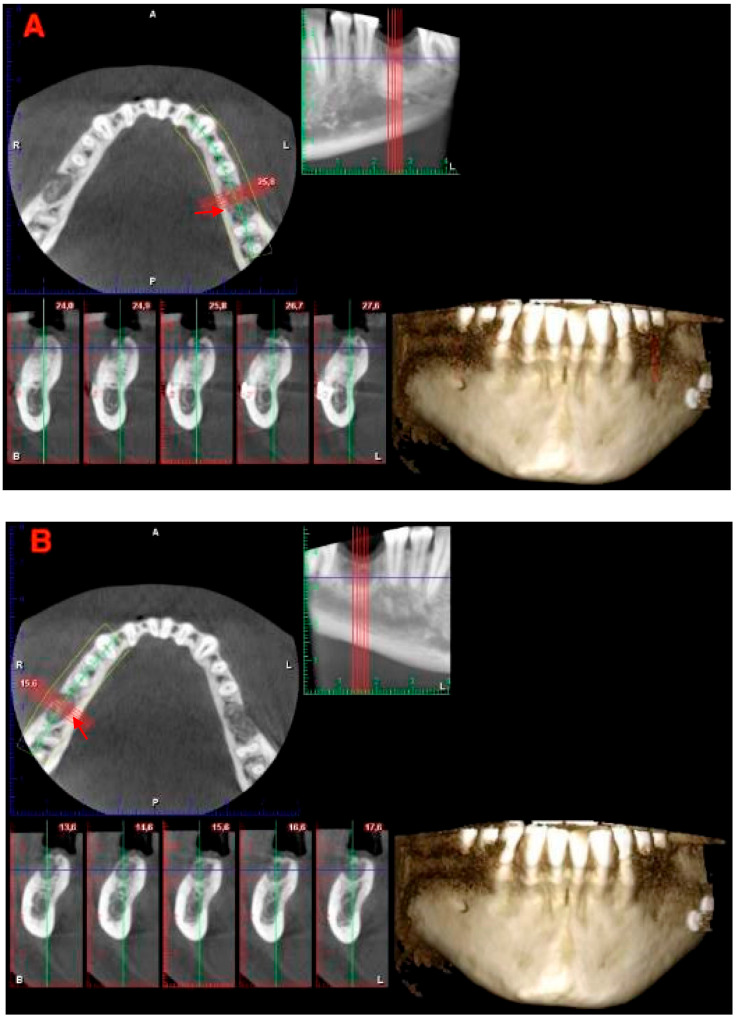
(A) CBCT at 6 months, 3.6. (B) CBCT at 6 months, 4.6. A good profile of the alveolar ridges seems to be maintained. Red arrows point the profile of the alveolar ridge.
